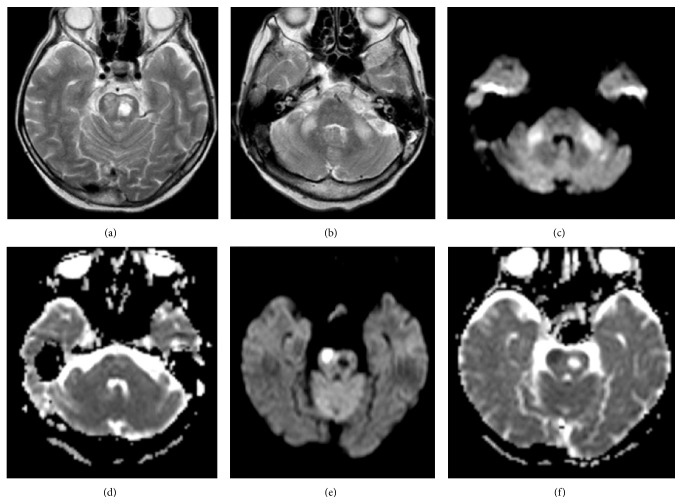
3 months after left paramedian acute pontine infarction. Axial T2WI (a) hyperintense signal in pons due to chronic infarct. Axial T2WI (b) bilateral and symmetrical hyperintense signal in MCPs due to WD. Axial DWI (c) and ADC map (d) shows hyperintense signal. Axial DWI (e) and ADC map (f) shows restricted diffusion due to new acute infarct in the right side of the pons.
